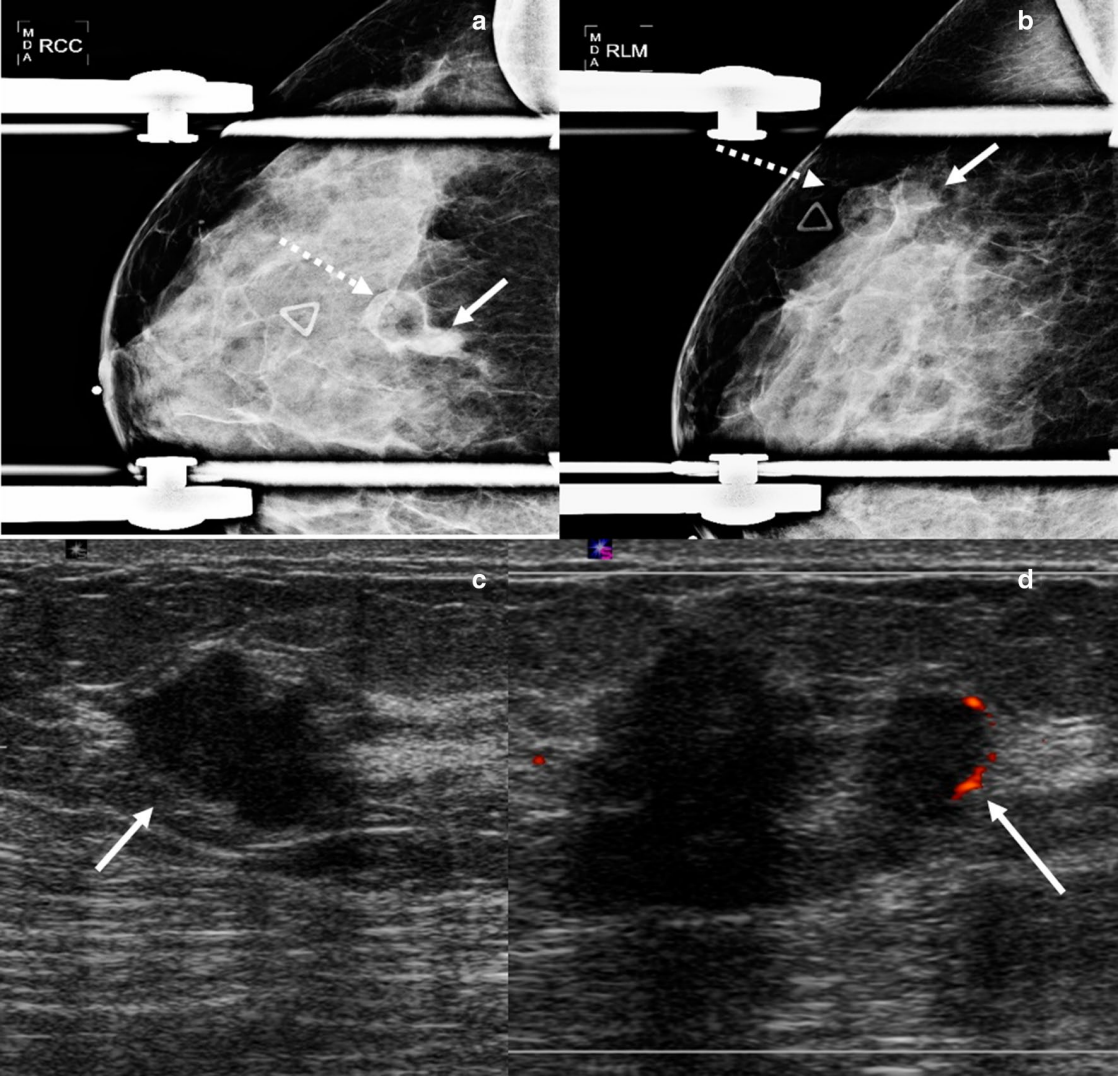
Tubular adenoma. A 36-year-old woman presented with a palpable breast mass. Spot cradiocaudal and lateral mammogram (a, b) shows a high-density irregular mass (solid arrow), adjacent to an incidental oil cyst (dotted arrow). Grayscale (c) and power Doppler ultrasound (d) images show an irregular hypoechoic mass with increased vascularity (arrows). Core needle biopsy showed tubular adenoma. The mass remained stable sonographically for 30 months and mammographically for 9 years
